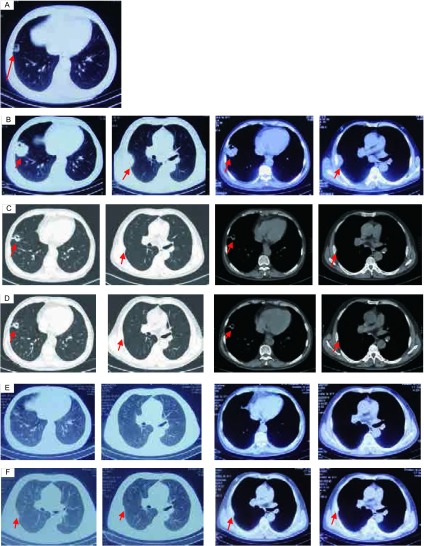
CT图像。A：患者发病5年前体检时肺CT；B：患者发病初始肺CT（2013年2月）；C：2个周期化疗后肺CT（2013年4月）；D：4个疗程化疗后肺CT（2013年5月）；E：6个疗程化疗+分子靶向治疗后肺CT（2013年7月）；F：分子靶向药物维持治疗2个月后肺CT（2013年9月）。 CT graphs. A: patient's pulmonary CT before 5 years; B: Patient's pulmonary CT at the beginning of the disease (February, 2013); C: patient's pulmonary CT after 2 cycles of chemotherapy (April, 2013); D Patient's pulmonary CT after 4 cycles of chemotherapy (May, 2013); E: Patient's pulmonary CT after 6 cycles of chemotherapy and TKI treatment (July, 2013); F: Patient's pulmonary CT by TKI as maintenance treatment after 2 months (September, 2013). CT: computed tomograpy; TKI: tyrosine kinase inhibitor.
